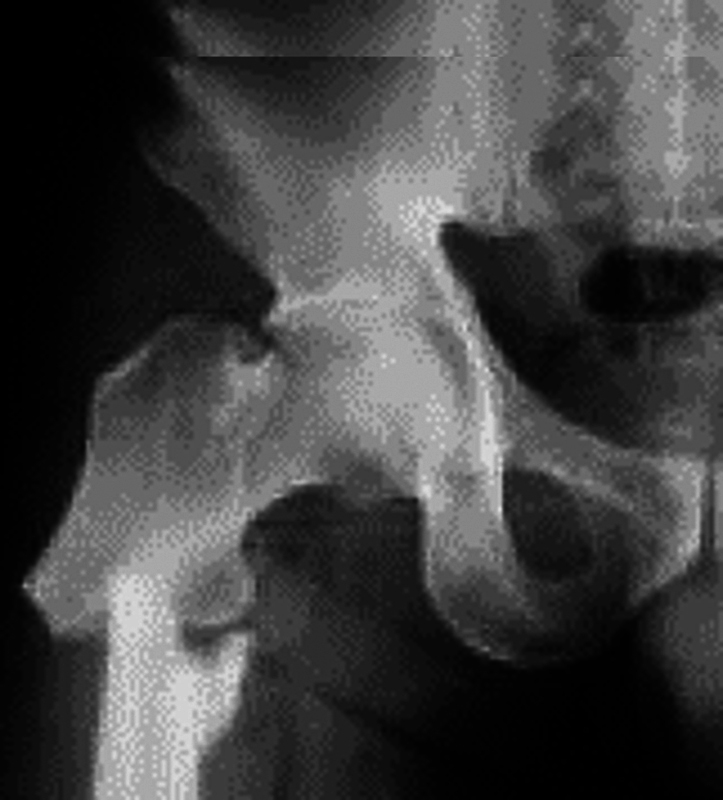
Radiograph showing an atypical right subtrochanteric femoral fracture in an elderly person after minimal trauma.
